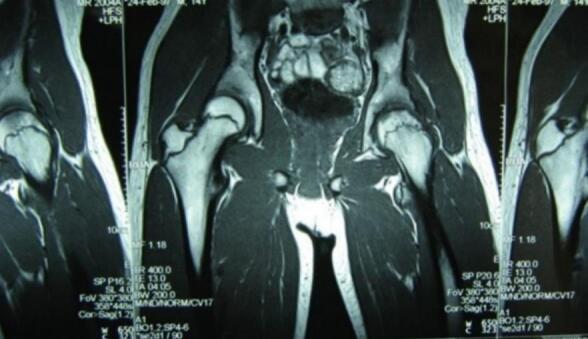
Pre-operative MRI (MRI show typical hypointense band at left femoral head, outlining the ischaemic segment beneath the articular surfaces) for patient I.
